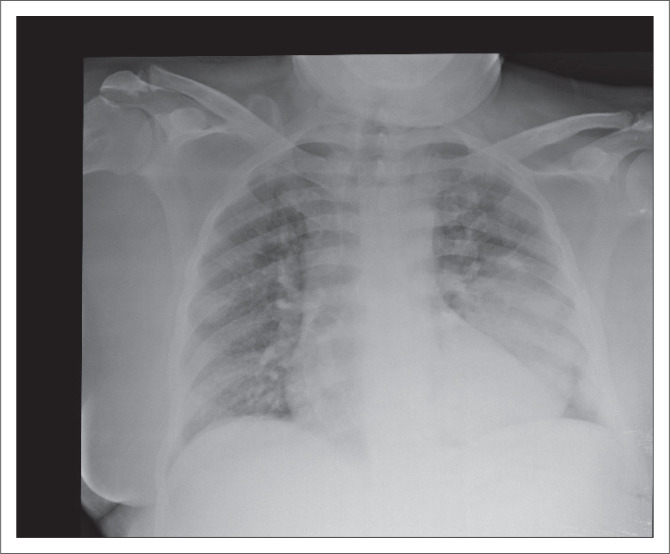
Chest radiograph showing a dense opacification in left lower lobe.
